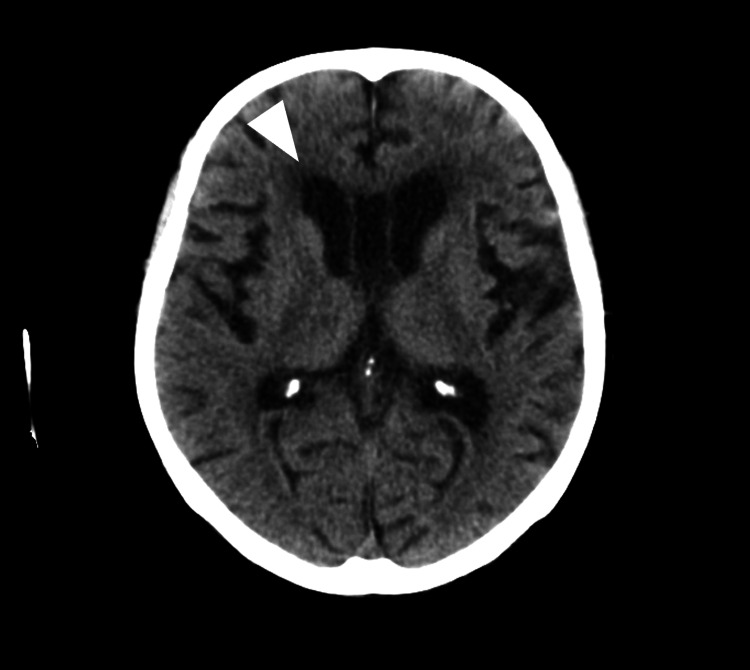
Head CT in the first episode No intracranial hemorrhage or mass effect was observed. The white arrow highlights a representative area of normal findings. CT: computed tomography
